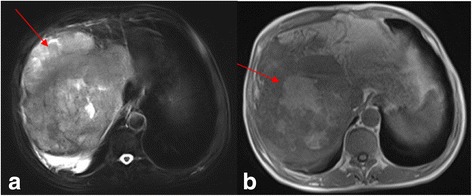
Transverse DCE-MRI of the abdomen shows lesions (arrows) in the right lobe of liver. A large mass in the right hepatic lobe is indicated by heterogeneous areas of high signal intensity (arrow) in (a) the T2-weighted image, and heterogeneous areas of low intensity signal (arrow) in (b) the T1-weighted image
